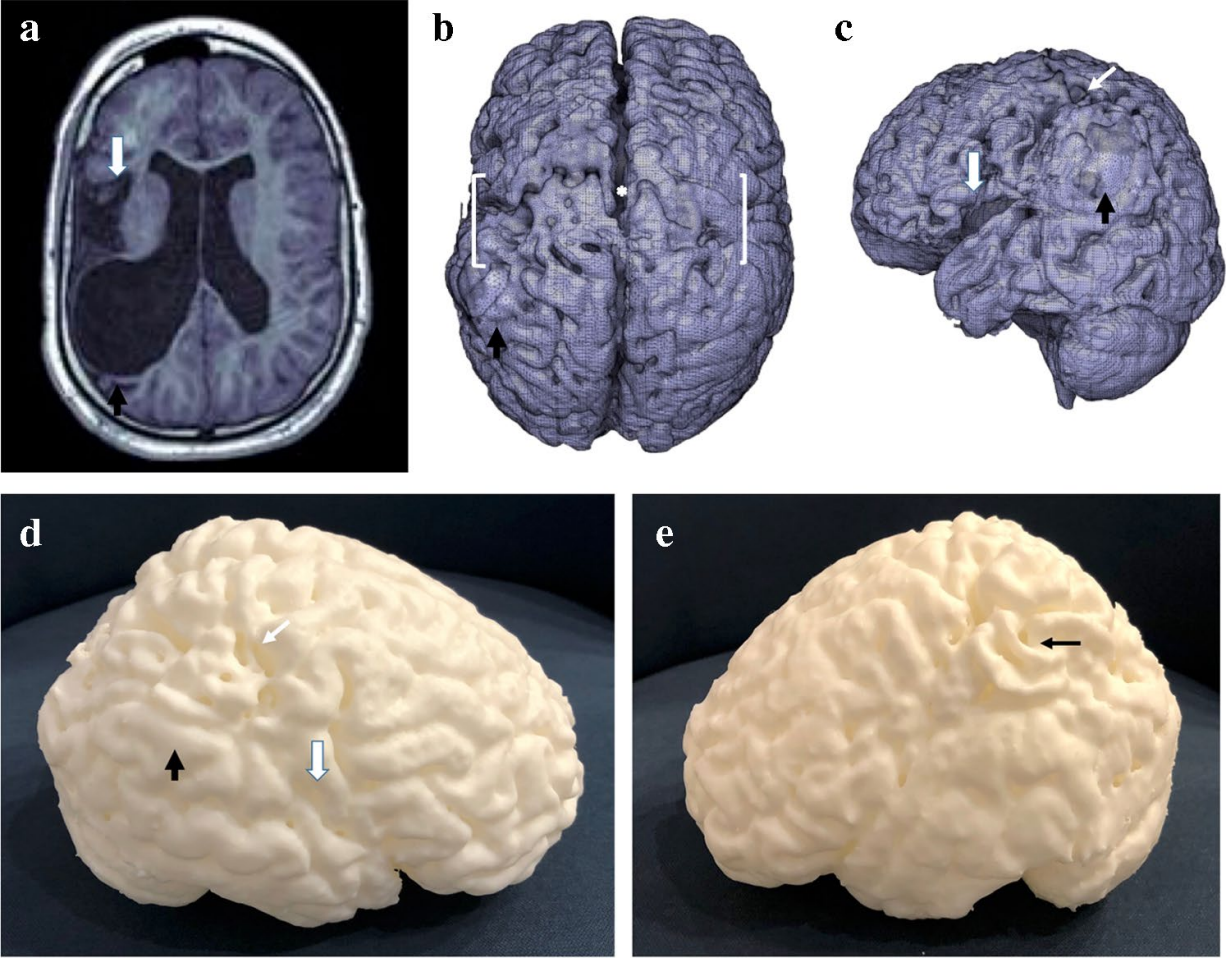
An 11-year-and-6-month-old boy with combined acute-profound and partial-prolonged hypoxic ischemic injury sustained perinatally. a Axial T1W magnetic resonance image shows focal asymmetric atrophy with a wide right peri-Sylvian fissure (*white arrow*). There is an expanded posterior body of the right lateral ventricle approximating the surface of the right parietal lobe (due to regional atrophy) and a very thin cortical ribbon (*black arrow*) accurately depicted in the 3-dimensional (D) images. b, c Vertex (b) and right-sided lateral (c) views of the 3-D mesh show bilateral asymmetric atrophy of the posterior peri-Sylvian and peri-Rolandic regions (*brackets*). The right is more affected than the left, although with an intact cortical rim, as evidenced by the expanded and smooth cortex overlying the occipital horn of the right lateral ventricle (*black arrow* in b). The smooth cortex is also seen in  (c)  (*black arrow*). Interhemispheric widening (*asterisk *in b) is a sign of atrophy. There is also atrophy of the superior and middle regions of the right peri-Sylvian fissure, which represents the watershed between all three major vessels supplying the brain (*small white arrows*) with associated widening of the peri-Sylvian fissure (*large white arrow* in c).  d, e Right (d) and left (e) lateral views of the 3-D print model accurately show the corresponding areas of involvement. There is widening of the peri-Rolandic (*small white arrow*) and peri-Sylvian (*large white arrow*) regions. The 3-D model accurately depicts the thin cortical mantle overlying the occipital horn of the right lateral ventricle (*black arrow* in d). The area of atrophy in (e) at the junction of the peri-Sylvian fissure, medial peri-Rolandic region and posterior intervascular watershed (*arrow*) is in keeping with a combined partial prolonged and acute profound hypoxic ischemic injury
